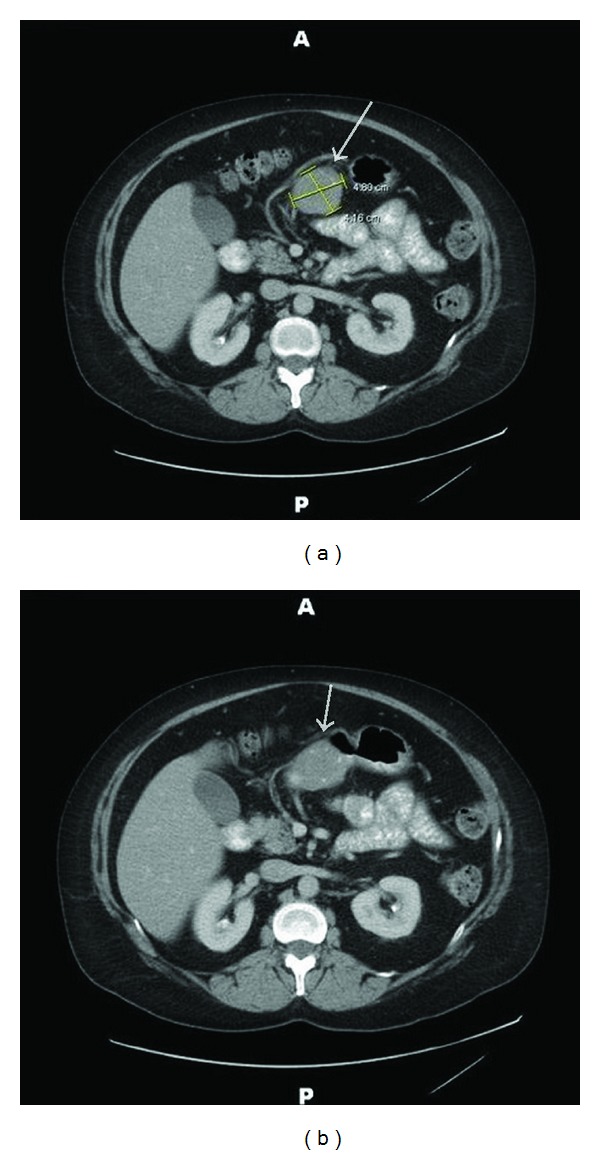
Contrast-enhanced CT showing a round, well-defined, and homogeneously enhancing mass arising from the antrum of the stomach.
